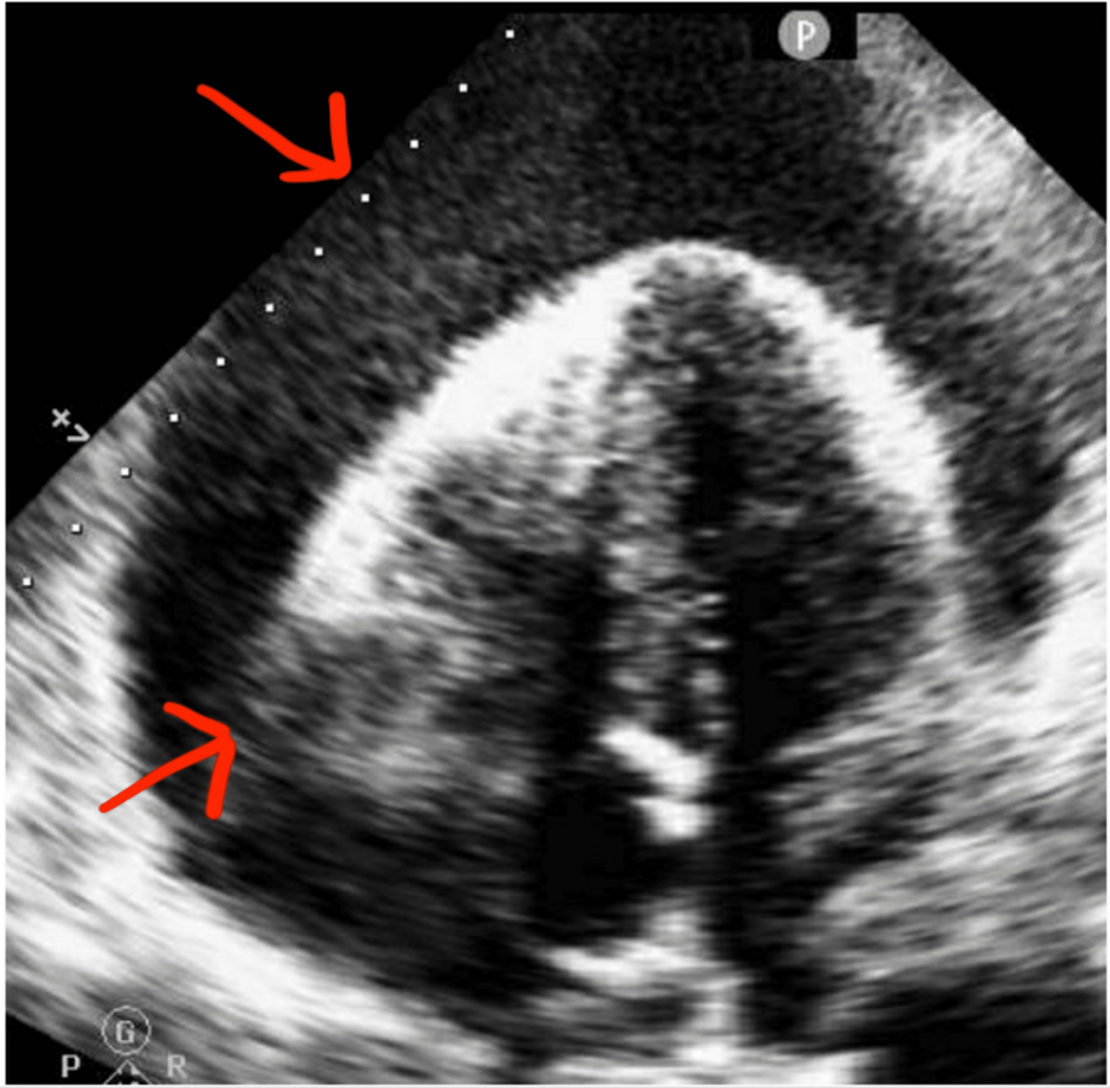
Echocardiogram showing large circumferential effusion up to 4.9 cm with right ventricular collapse.
